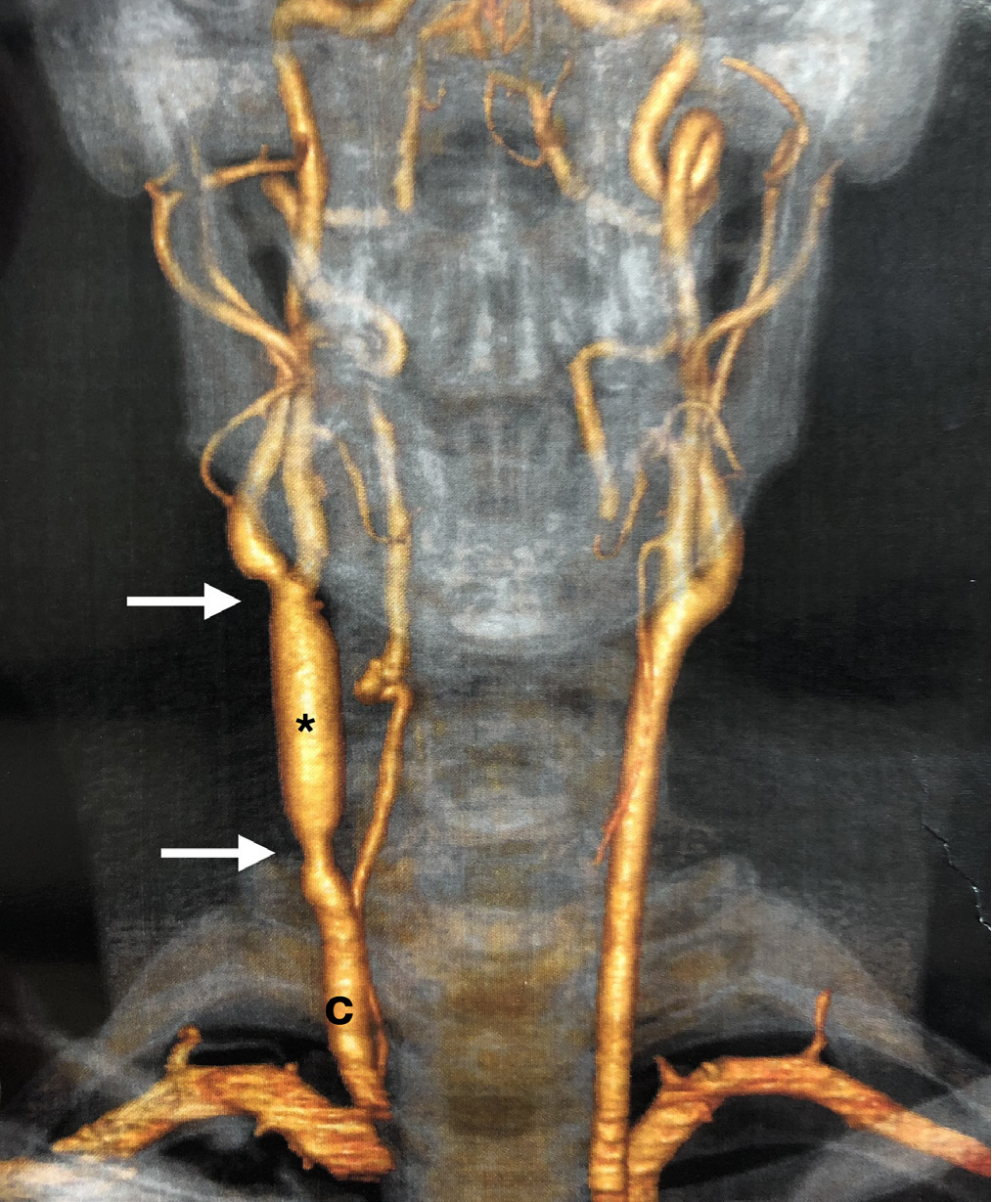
Computed tomography of the neck (volumetric rendering) in 18th postoperative month. * right external jugular vein graft; c: right common carotid, the arrows indicate the proximal and distal anastomoses.
